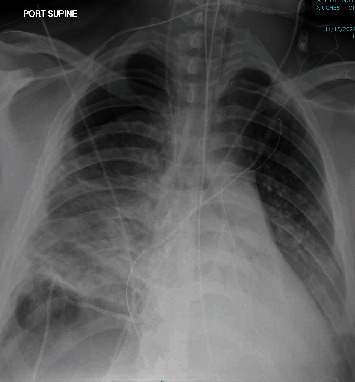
Chest X-ray after intubation, demonstrating a large right hydropneumothorax as a result of a bronchopleural fistula.
